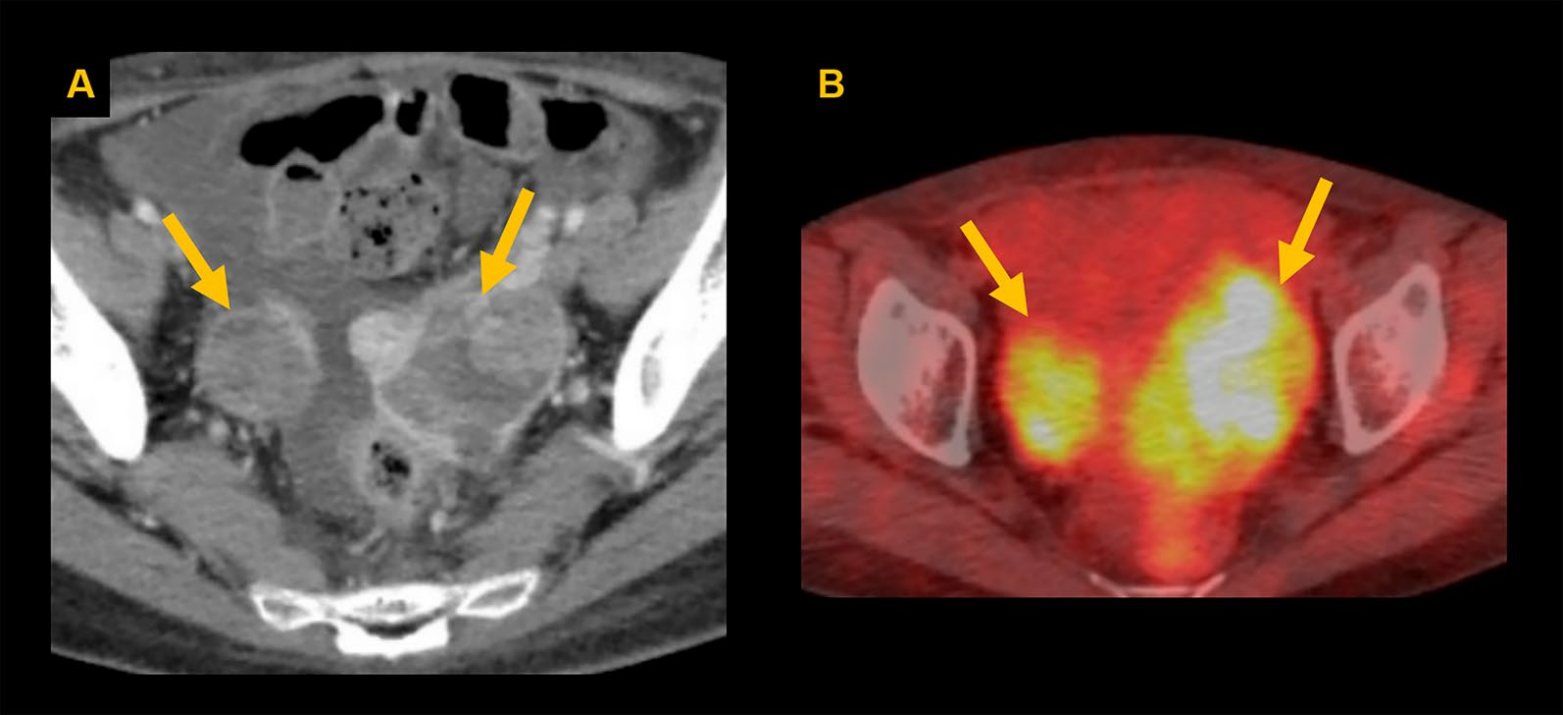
Early postoperative relapse. Contrast-enhanced CT images at 20 days postoperatively (A) shows bilateral pelvic masses (arrows) and FDG PET/CT images at 50 days postoperatively (B) confirmed their rapid growth with increased FDG uptake (arrows)
